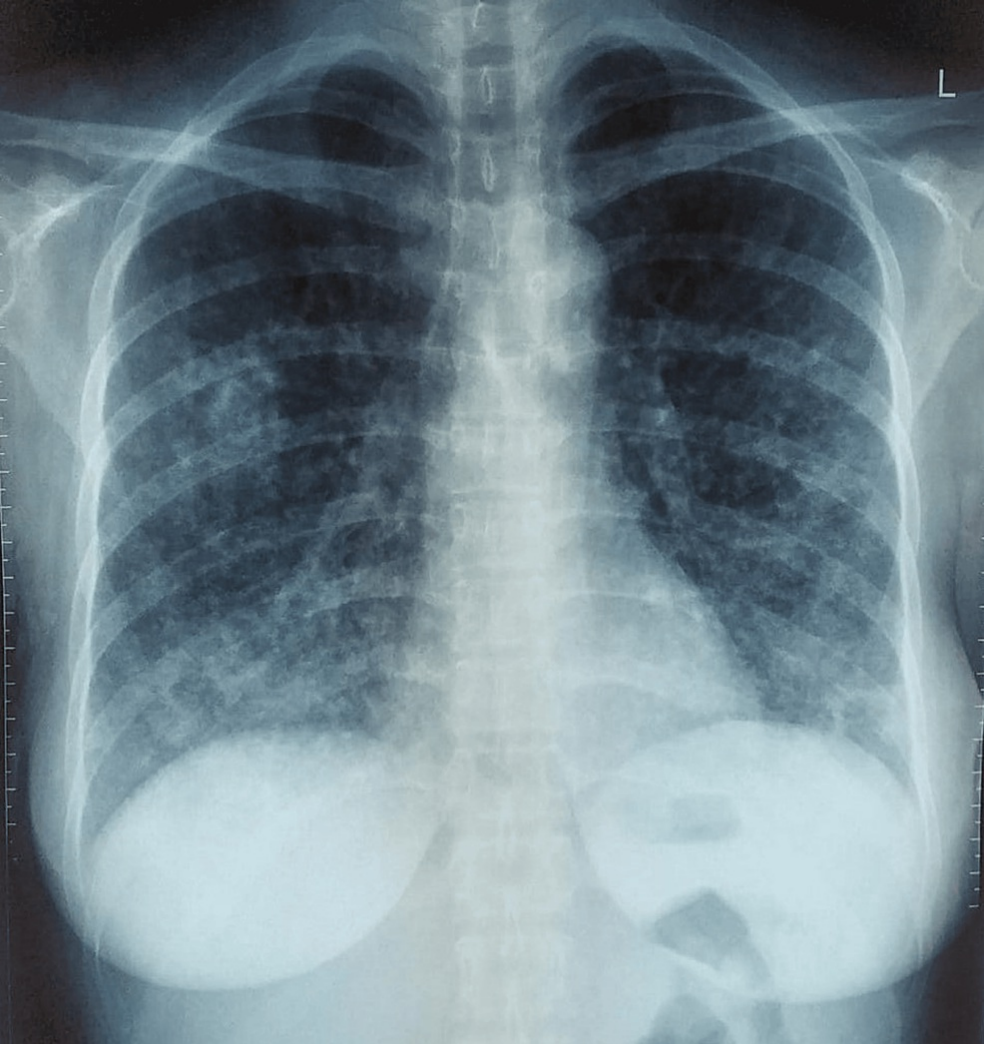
Chest X-ray in the primary hospital
